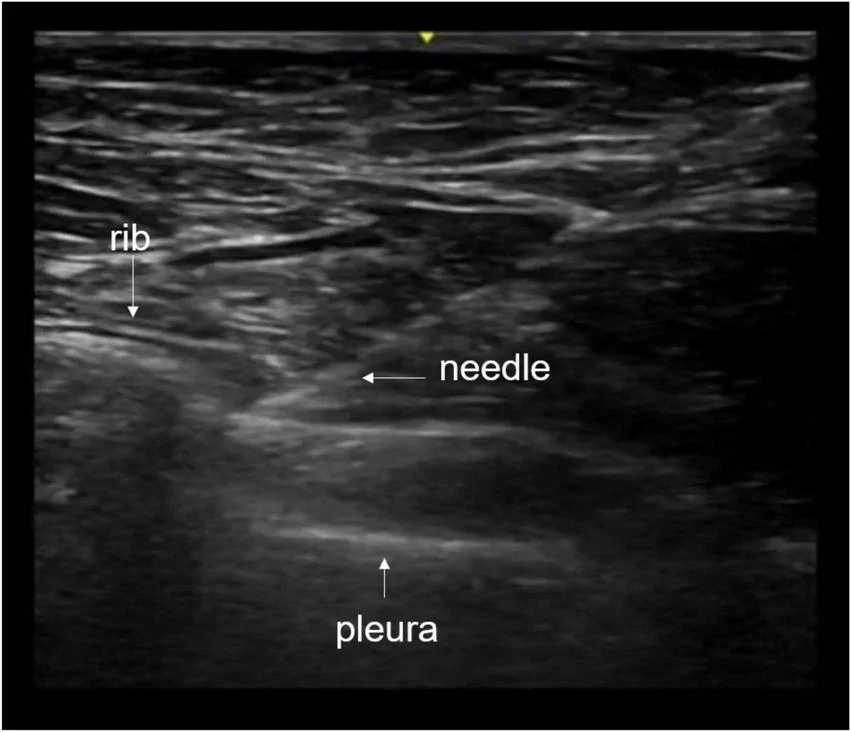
Ultrasound-guided intercostal nerve block.
